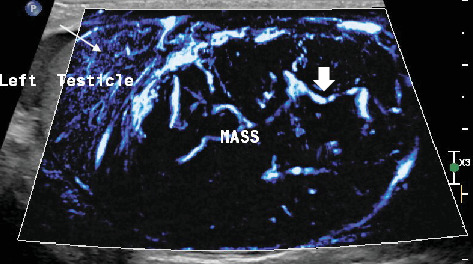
Marked intralesional vascularity and hyperemia on microflow imaging (MFI), a form of power Doppler imaging, in which vessels are seen as bright lines (thin long arrow). The image also depicts the typical curved and haphazard (short thick arrow) course of the vessels.
